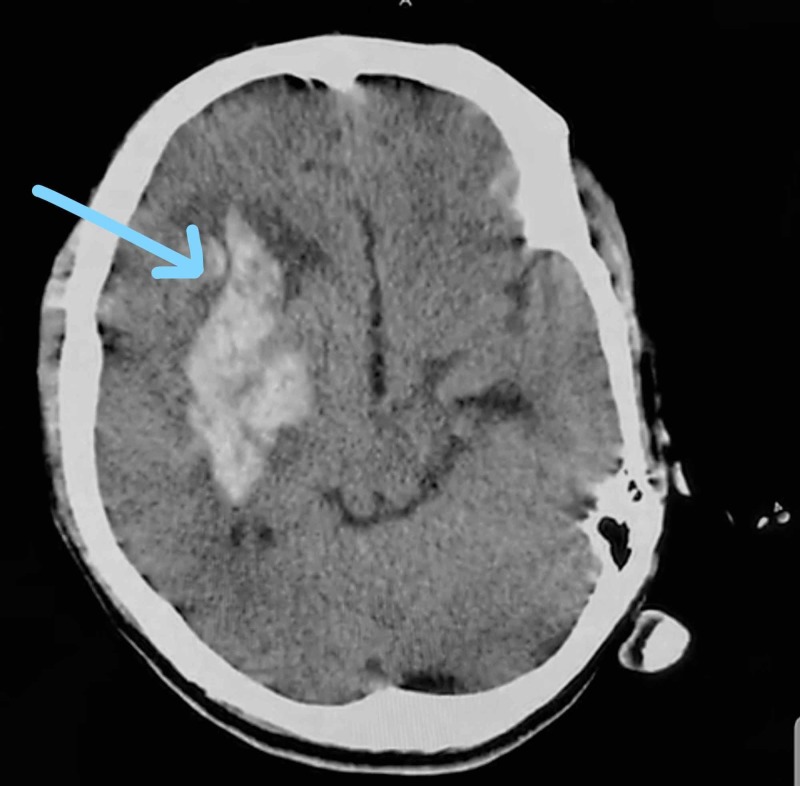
Head CT plain showing right large gangliocapsular hemorrhage.
